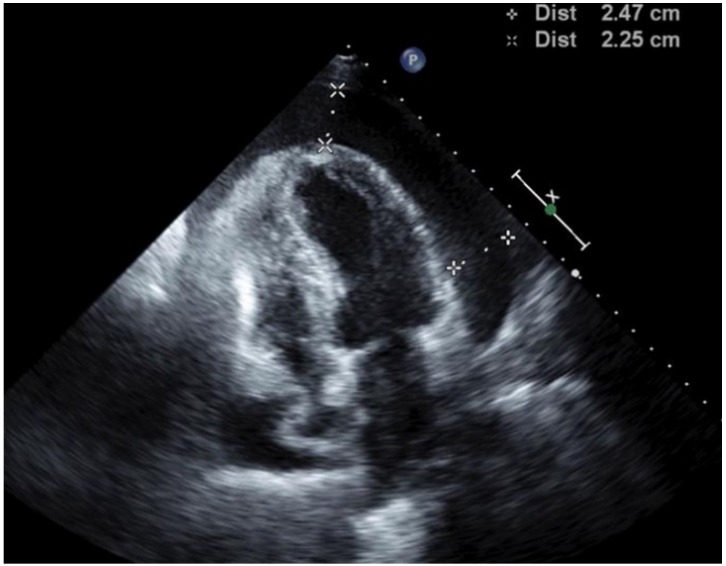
Transthoracic echocardiogram. Large pericardial effusion, right atrial and ventricular diastolic collapse consistent with cardiac tamponade.
